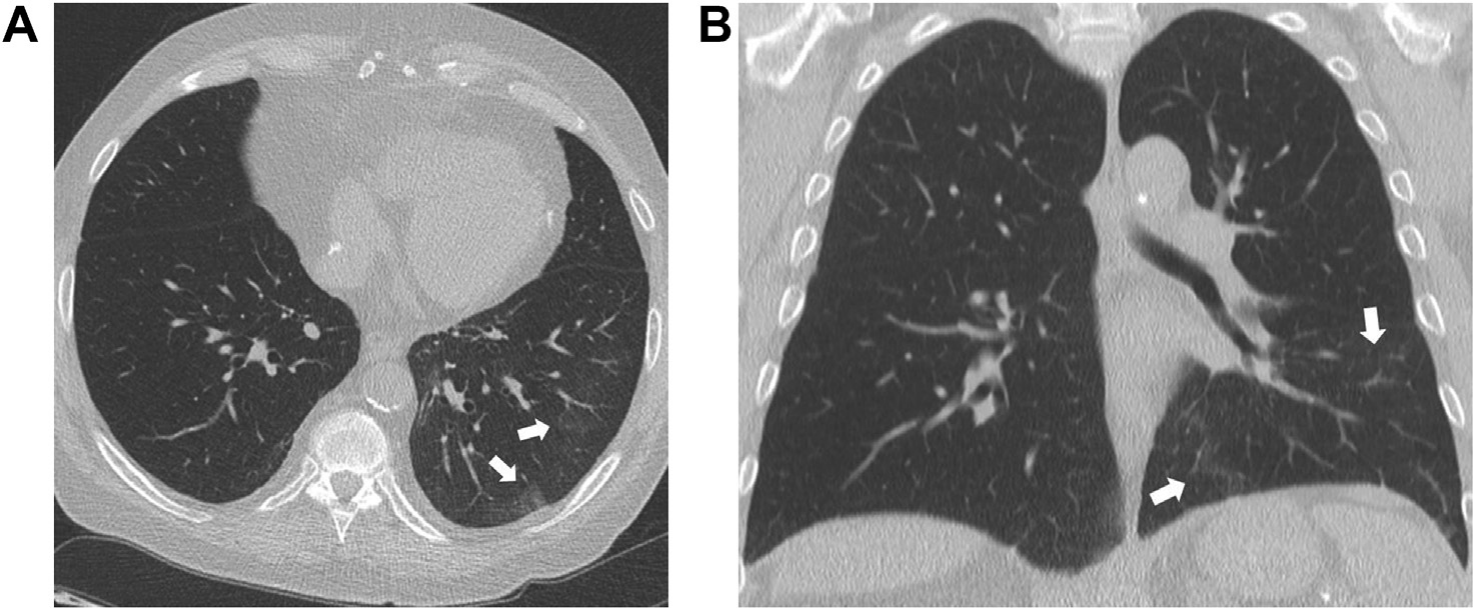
The second CT-scan on day 5 after hospitalization demonstrated already a significant decrease of ground glass opacities (GGO) in the left lower lobe (Figure 2A, transverse plane, *arrows*). No new GGO or consolidations were present compared to the initial CT scan. In addition, there was no pleural effusion or mediastinal lymphadenopathy (Figure 2B, coronal plane).
